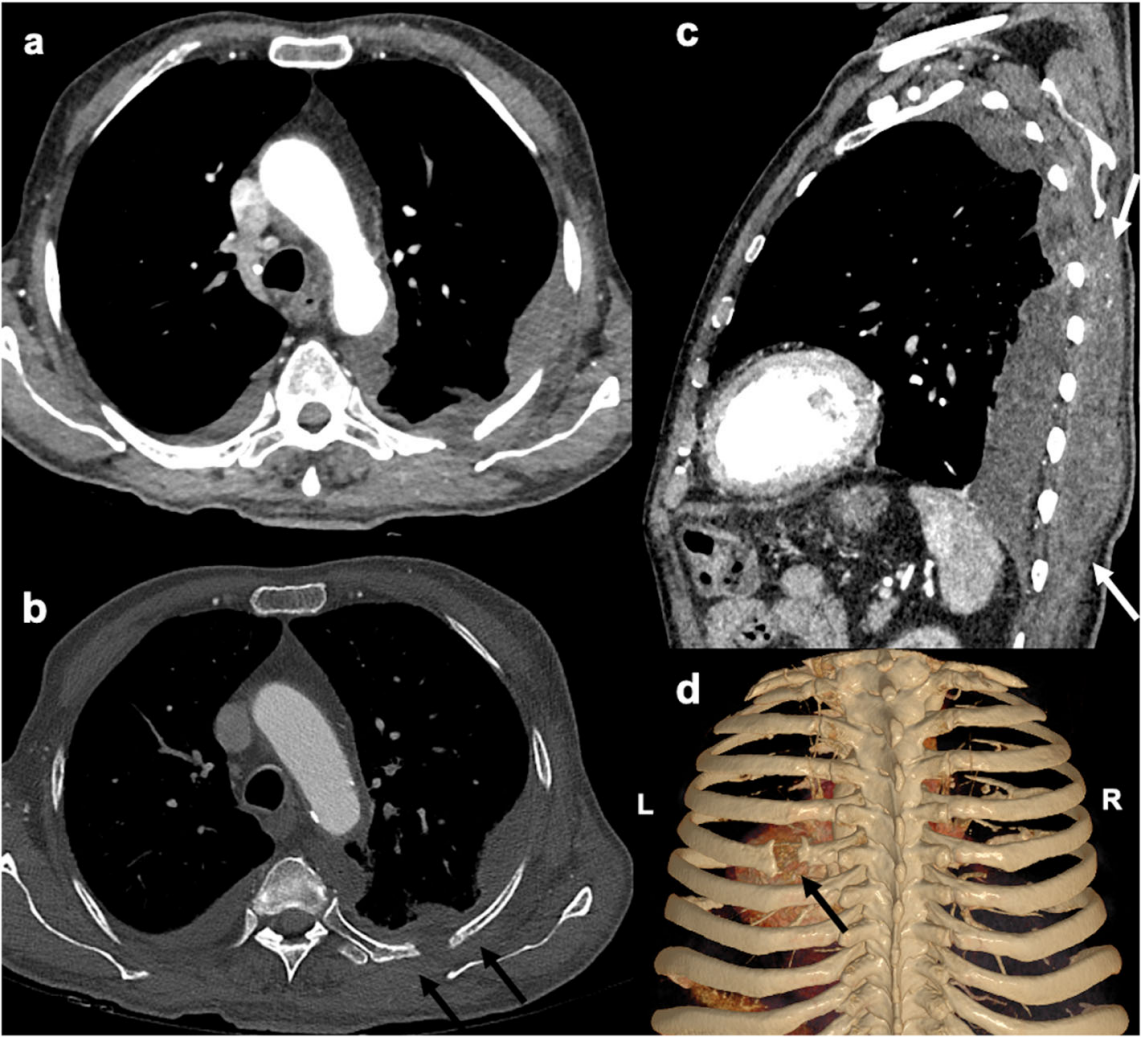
Contrast enhanced computed tomography (CECT) scan images showing rib osteomyelitis with empyema necessitans. (a, b) Axial images in (a) mediastinal, (b) bone windows showing left sided loculated pleural effusion with mild right sided pleural effusion. Black arrows in b show the defect in the involved rib with sclerotic changes in the remaining rib bone marrow. c Sagittal image shows the pleural fluid extending into the chest wall till subcutaneous tissues plane (white arrows). d Volume rendered image shows the rib defect with associated periosteal reaction (black arrow)
